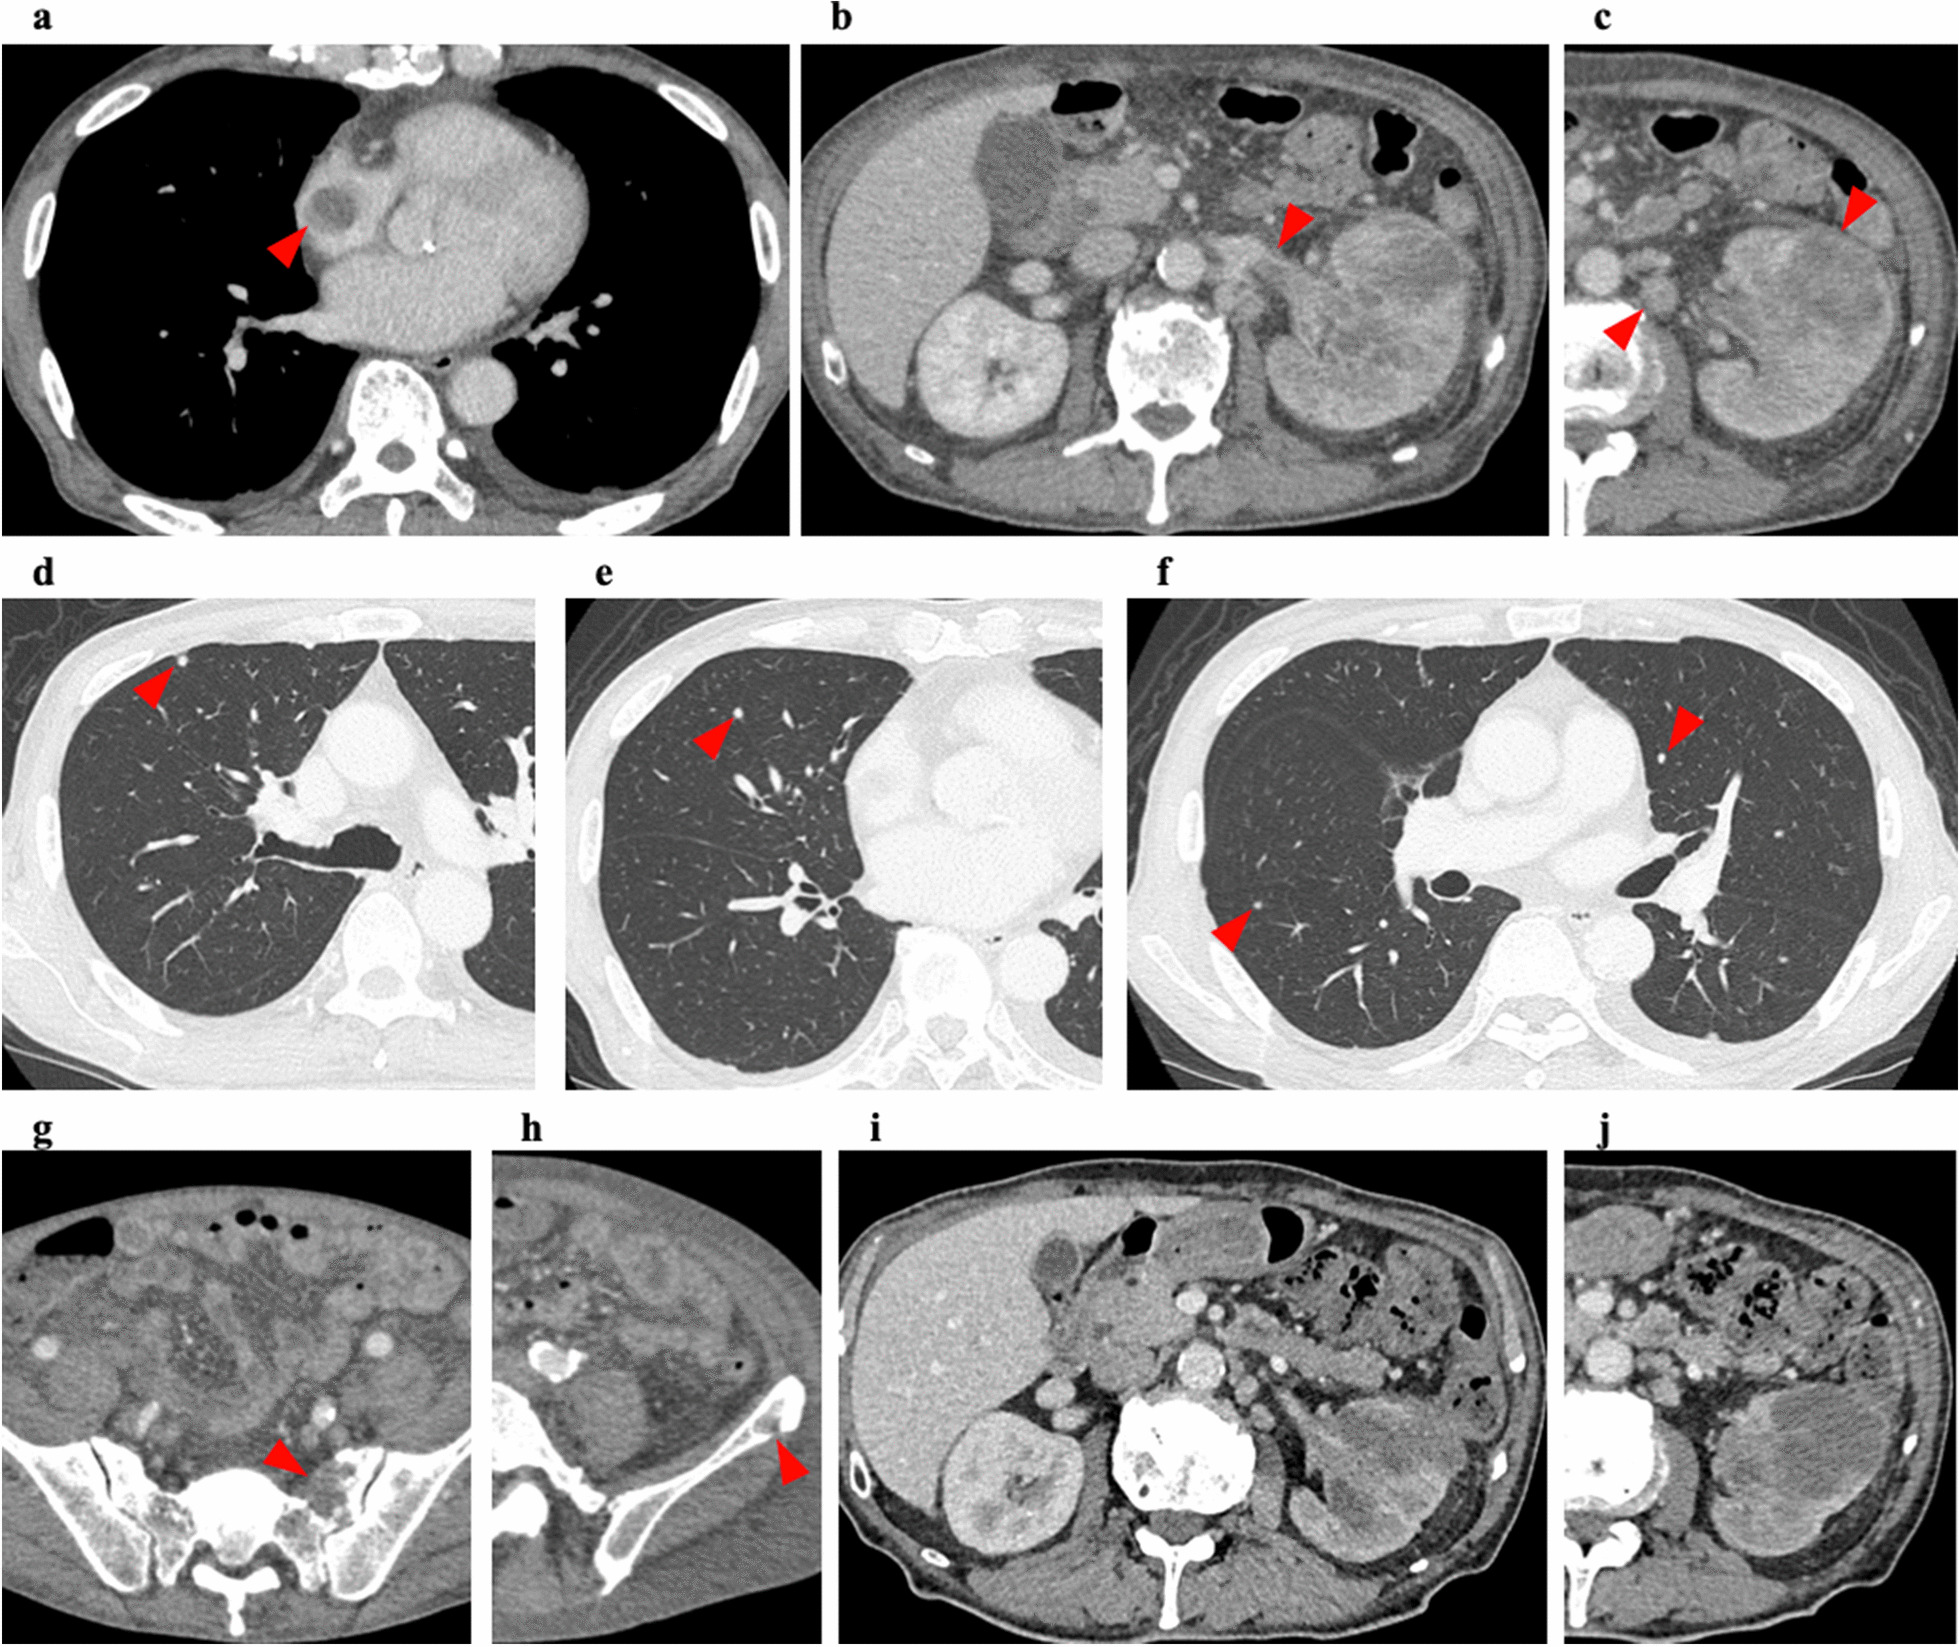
Chest and abdominal CT scan. Abnormal findings are indicated by arrowheads. a 18 mm mass found in the right atrium. b Tumor thrombus extending into the renal vein. The right kidney represents a normal and healthy control. c Heterogeneously enhanced left renal tumor. Para-aortic lymphadenopathy is also observed. d–f Multiple small lung nodules suspected of metastases. g, h Multiple bone metastases were observed after the second administration of ipilimumab and nivolumab. i Left renal vein tumor thrombus almost disappears following immunotherapy. j Left renal tumor and para-aortic lymphadenopathy remain reduced with continued immunotherapy
